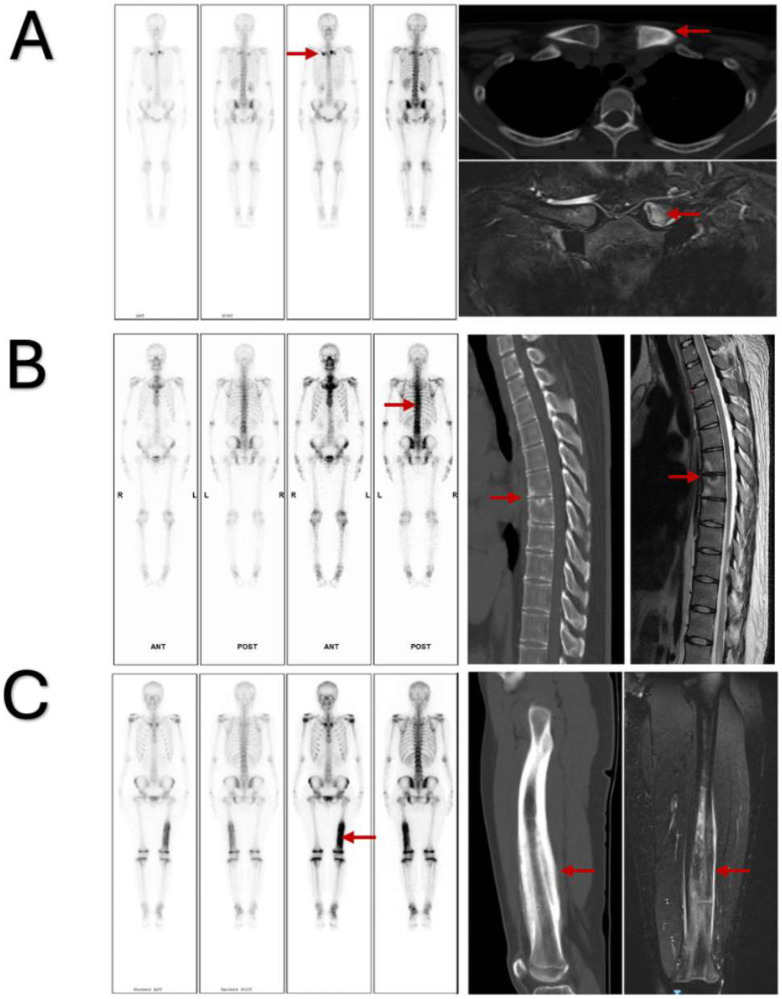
Representative whole-body bone scintigraphy, computed tomography, and magnetic resonance imaging findings in patients with SAPHO syndrome. (A): Cluster 1 (typical SAPHO syndrome); (B): Cluster 2 (axial SAPHO syndrome); (C): Cluster 3 (peripheral SAPHO syndrome).
